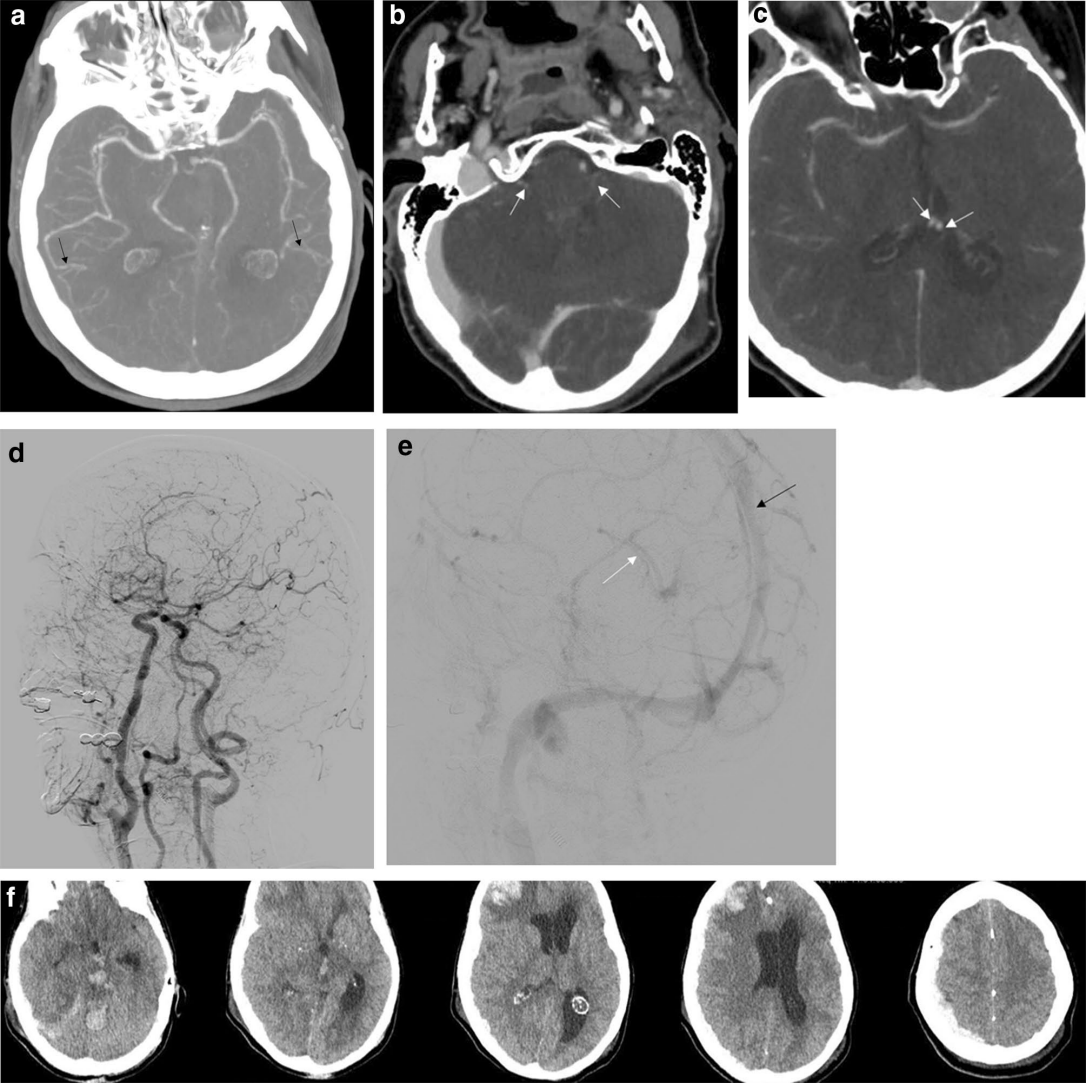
(a) Axial CTA-MPR (arterial phase) reveals good patency to all arterial intracranial vessels (including M4 segments-black arrows). (b) Axial CTA at the same patient (60 s phase), shows opacification of both superior petrosal veins (white arrows). (c) Axial CTA at the same patient (60 s phase), shows opacification of both internal cerebral veins (white arrows). (d) Digital Subtraction Angiography at the same patient in arterial phase (LAO oblique view), reveals good flow in all proximal and distal intracranial arteries of the anterior and posterior circulation. (e) Digital Subtraction Angiography at the same patient in venous phase (LAO oblique view), shows that superficial (black arrow) and deep venous system (white arrow) is patent. (f) Axial plain brain CT at different levels, shows adequate differentiation of gray-white matter and no radiologic signs of brain death, 15 days following the initial angiographic check of the same patient.
